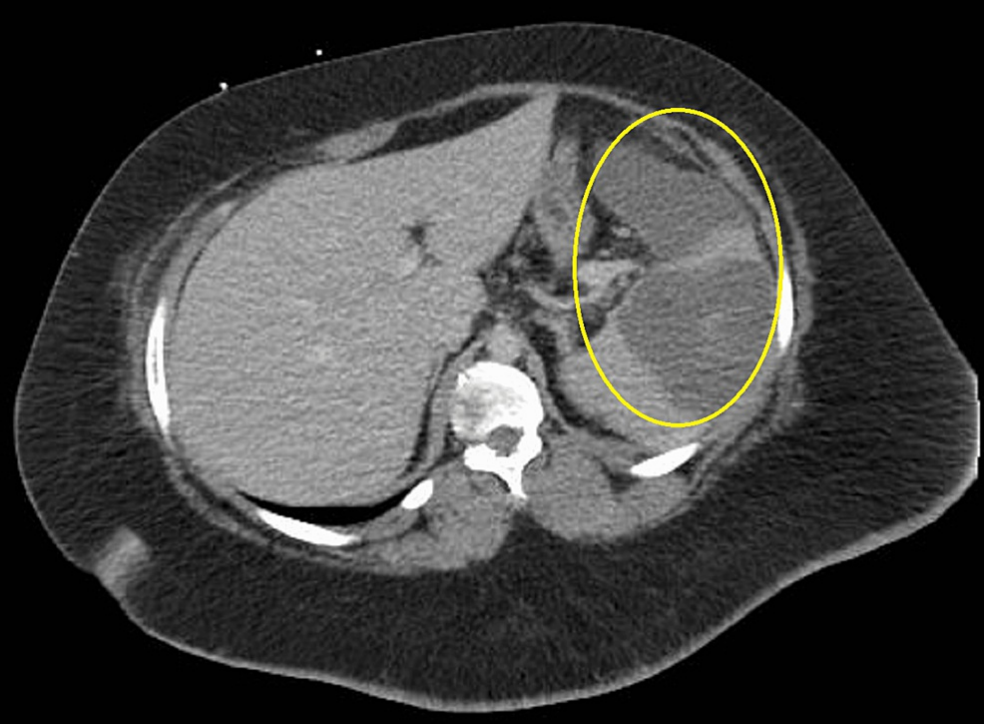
Axial section of CT scan of the abdomen showing the expansion of the lesion in the spleen with multiple areas of decreased attenuation CT, computed tomography
